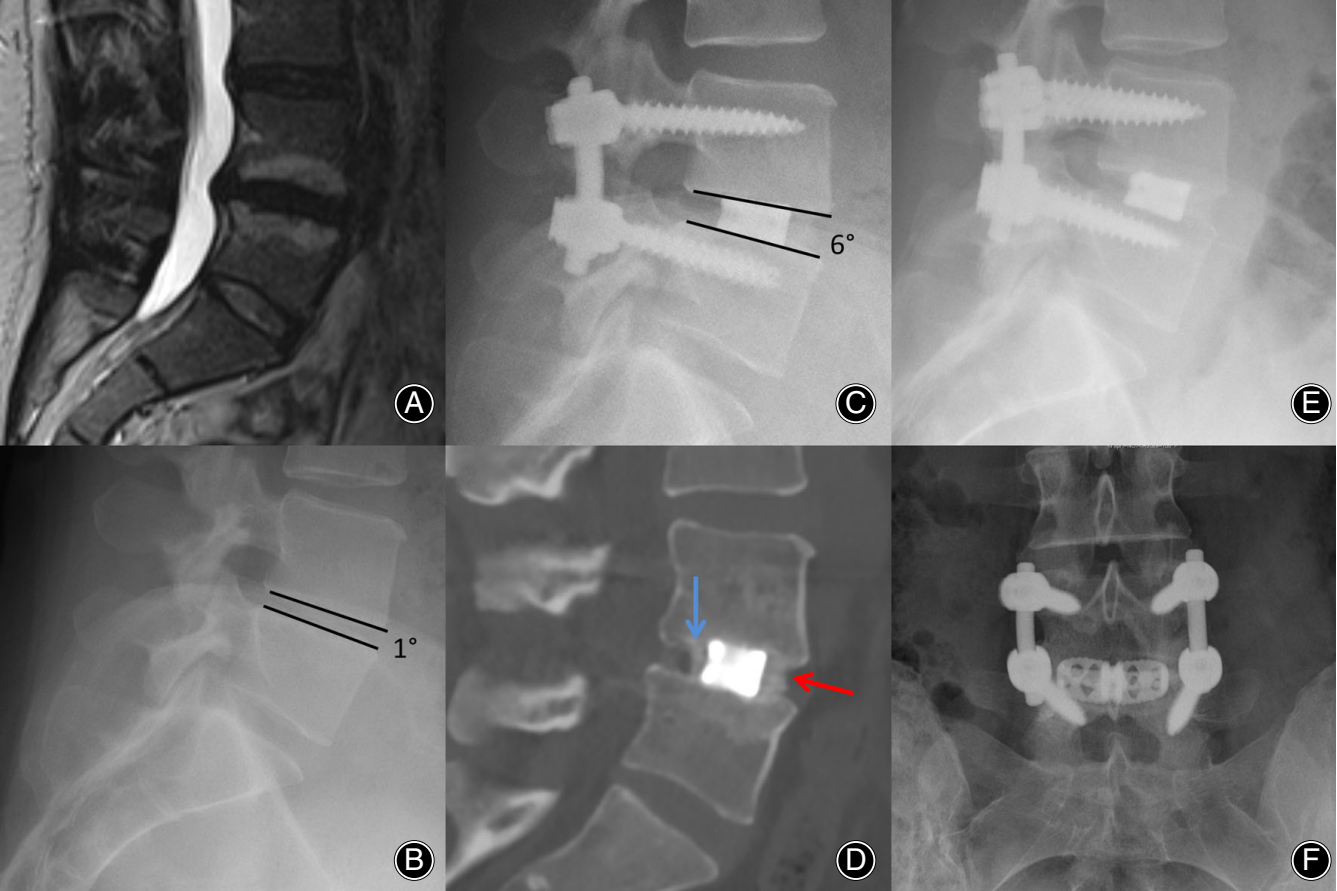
(A) Preoperative MRI Scan of a 36‐year old woman with severe osteochondrosis L 4/5 and disc herniation. Clinically, she complains of therapy‐resistant back pain and radiation to her left leg. (B) Preoperative lateral X‐ray shows a segmental lordosis L 4/5 of 1°. (C) The follow‐up 5 days after surgery with mono‐segmental fusion and implantation of a lordotic cage shows an improved segmental lordosis of 6° (increase of 5°). (D) The CT control after 1 year shows a stable bony fusion posterior to the cage (blue arrow) and anterior to the cage (red arrow). (E, F) The final X‐ray control 2 years after surgery demonstrates a significantly increasing bony fusion compared to (C) and (D).
